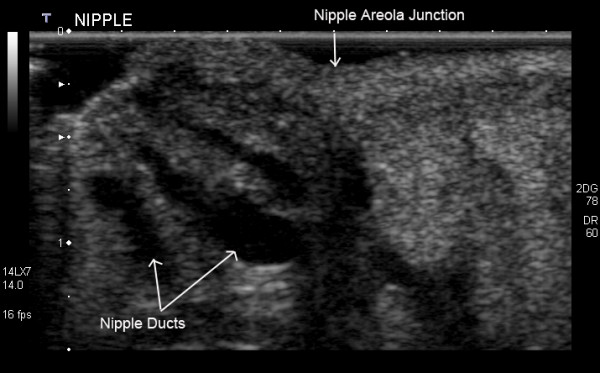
Ultrasound image of the nipple of a lactating breast. Ducts can be observed in the nipple as hypoechoic tubular structures. Visualization depends upon both the size of the duct and the resolution of the ultrasound equipment.
